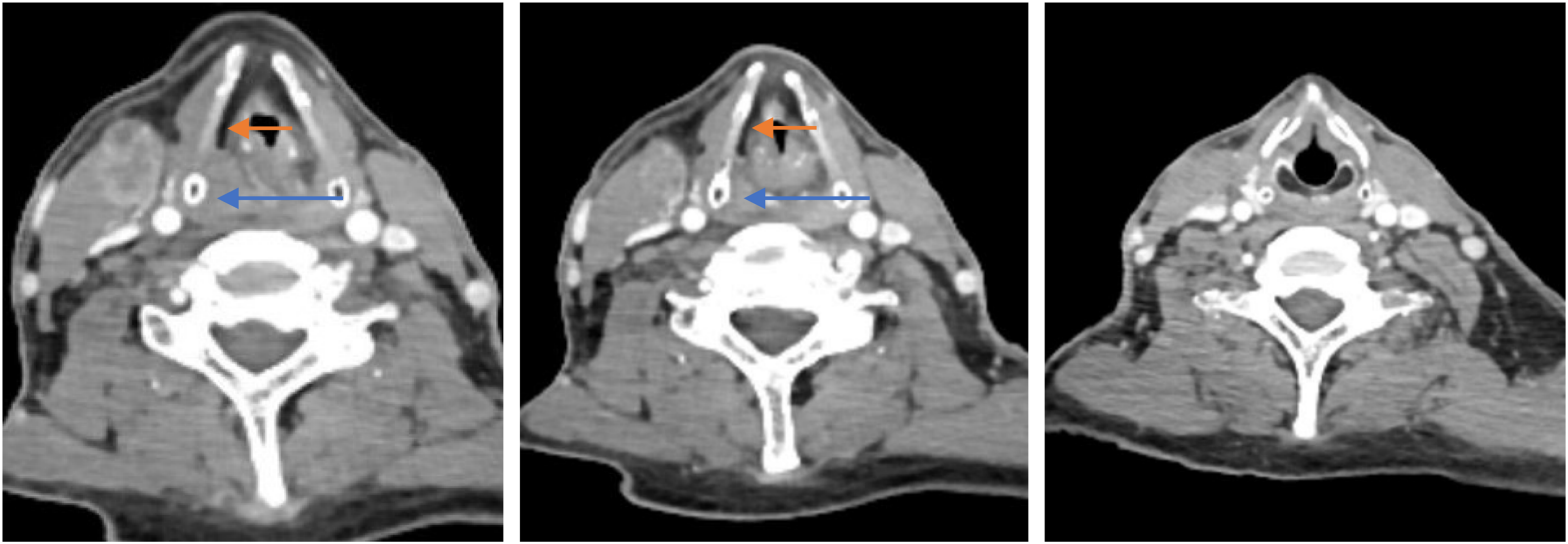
Preoperative contrast CT with contrast of one patient who was treated with the paraglottic approach. The paraglottic space was identified not completely invaded and the esophagus was not involved. The blue arrow indicates the tumor. The red arrow indicates the paraglottic space.
